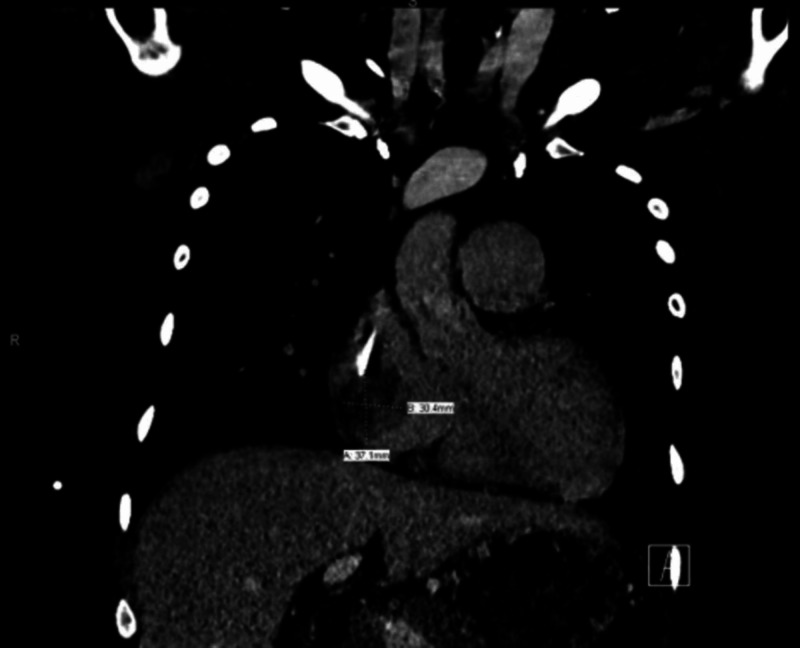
Coronal view of contrast-enhanced CT of the chest The image shows right atrial filling defect measuring 3.7 x 3.0 cm CT: computed tomography
